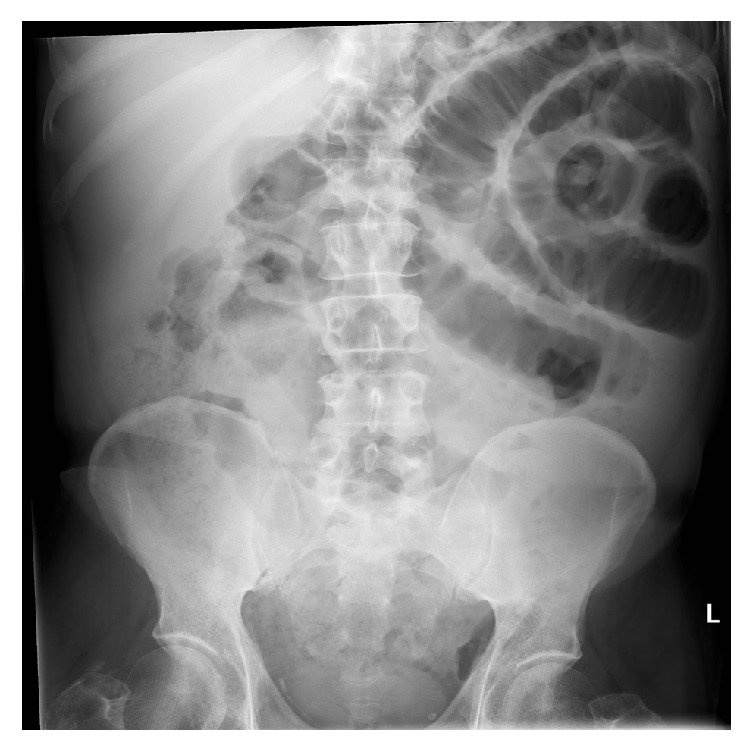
Plain film of abdomen showing dilated small bowel loops. March 2011.
